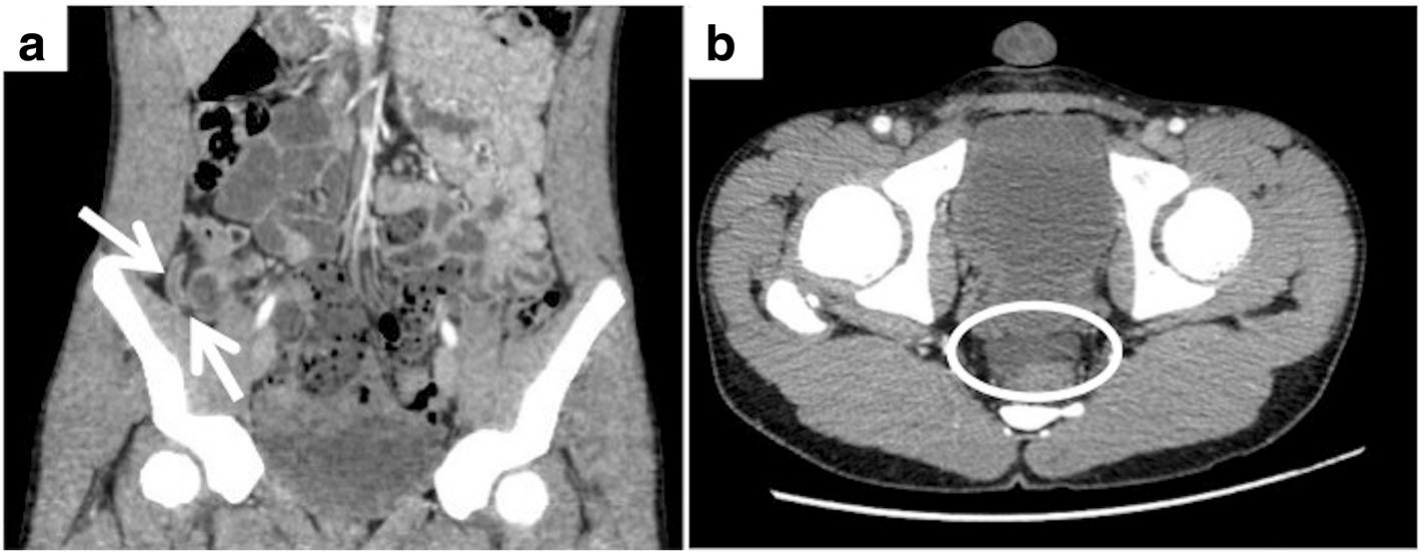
Enhanced computed tomography scan (CT) on admission. a CT shows a slightly enhanced and thickened appendiceal wall (arrow). Although the ileum is slightly dilated, intestinal obstruction cannot be detected. b CT also shows ascites at the recto-vesical pouch
